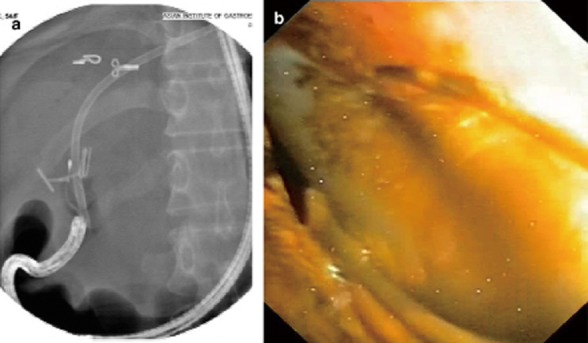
Retrieval of a migrated plastic stent using a basket catheter with the prototype cholangioscope. (a) X-ray image. (b) Endoscopic image.
